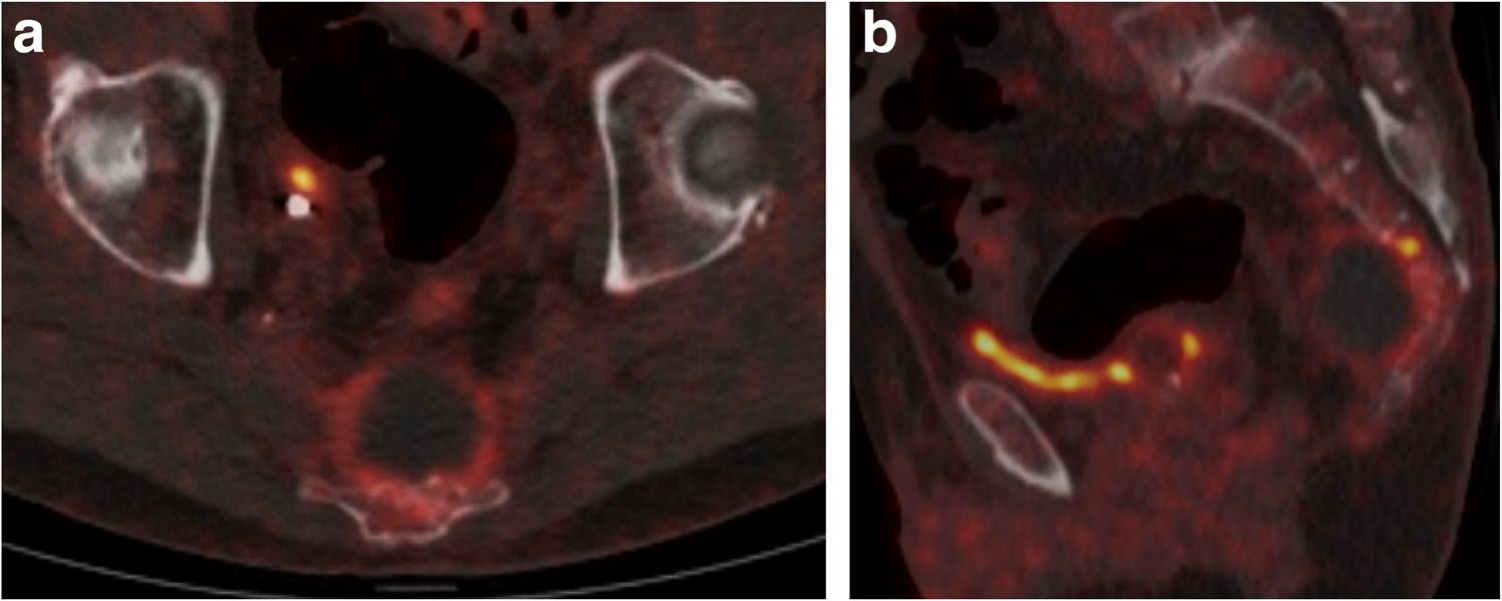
Fused ^18^F-FDG PET-CT images of the sacrococcygeal tumor at baseline. a Axial image shows a peripheral rim of FDG uptake in the soft tissue mass. b Sagittal image shows an osteolytic tumor with moderately increased metabolic activity in the rim of the soft tissue component. There is discrete cortical destruction at level S4 with a focus of high FDG uptake within the marrow
